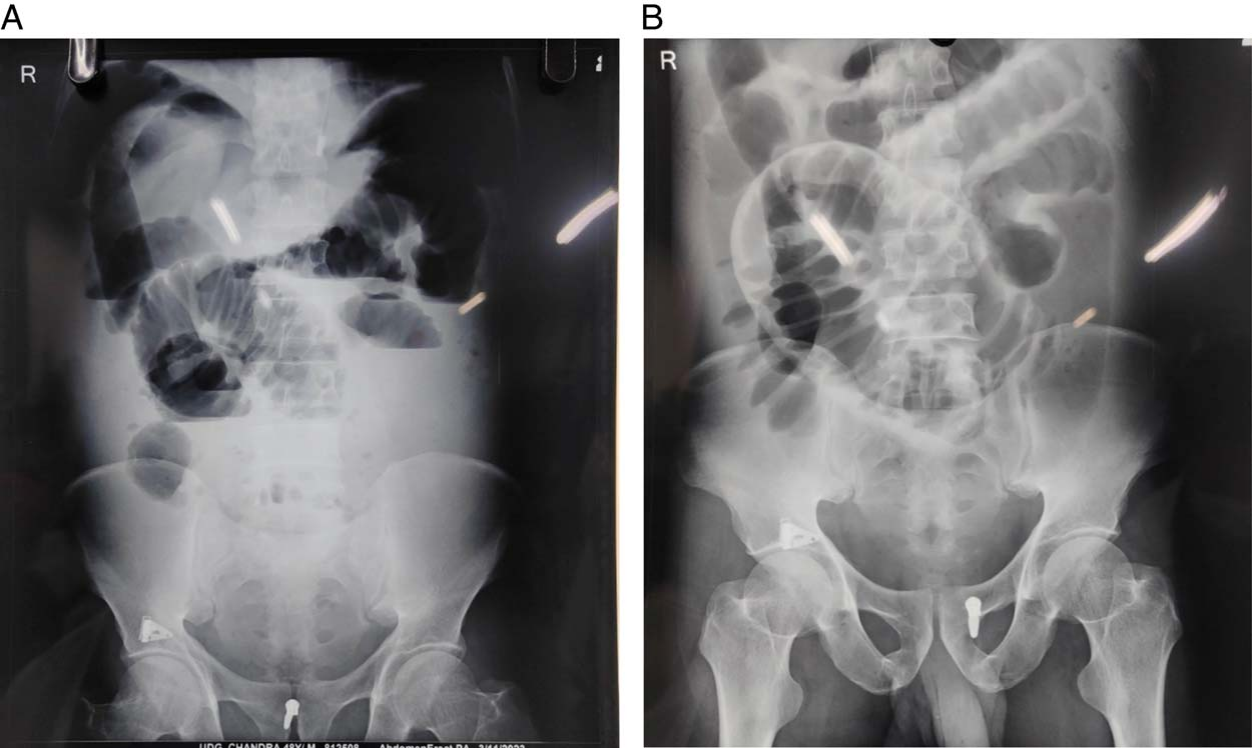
(A) Erect radiograph abdomen showing air-fluid level suggesting small bowel obstruction. (B) Supine radiograph abdomen showing distended small bowel loops involving whole small bowel.
